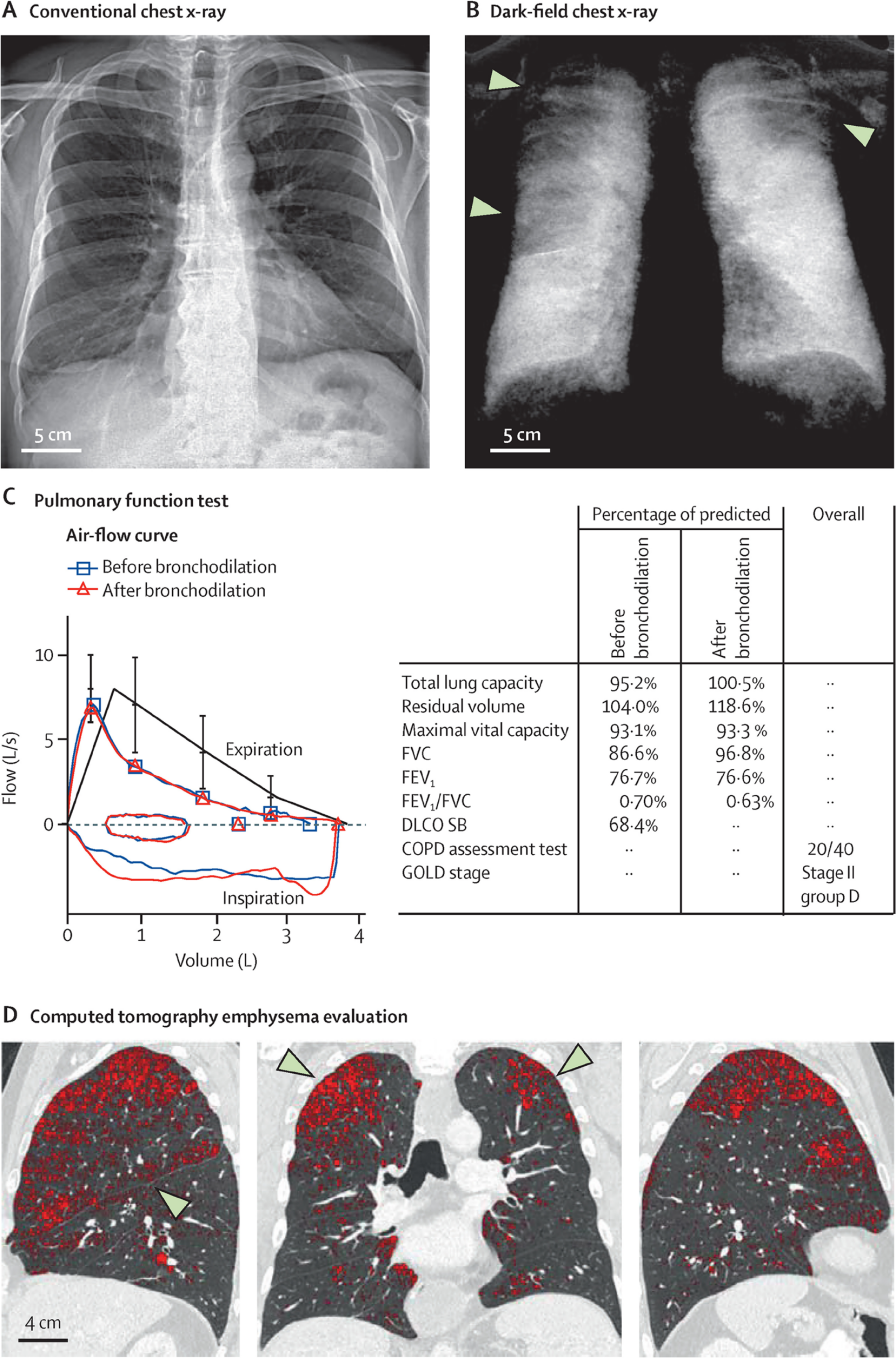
Conventional chest X‐ray and dark‐field X‐ray image (a/b) in a subject with chronic obstructive pulmonary disease with results from spirometry (c) and CT images (d). Emphysematous regions are readily apparent in the dark‐field X‐ray image but hard to see on conventional chest X‐ray. Reproduced from [146], published under the terms of a Creative Commons Attribution License, https://creativecommons.org/licenses/by/4.0/. COPD, chronic obstructive pulmonary disease; DLCO SB, diffusing capacity of the lung for carbon monoxide measured in a single breath; FEV1, forced expiratory volume in 1 s; FVC, forced vital capacity; GOLD, global initiative for chronic obstructive lung disease.
